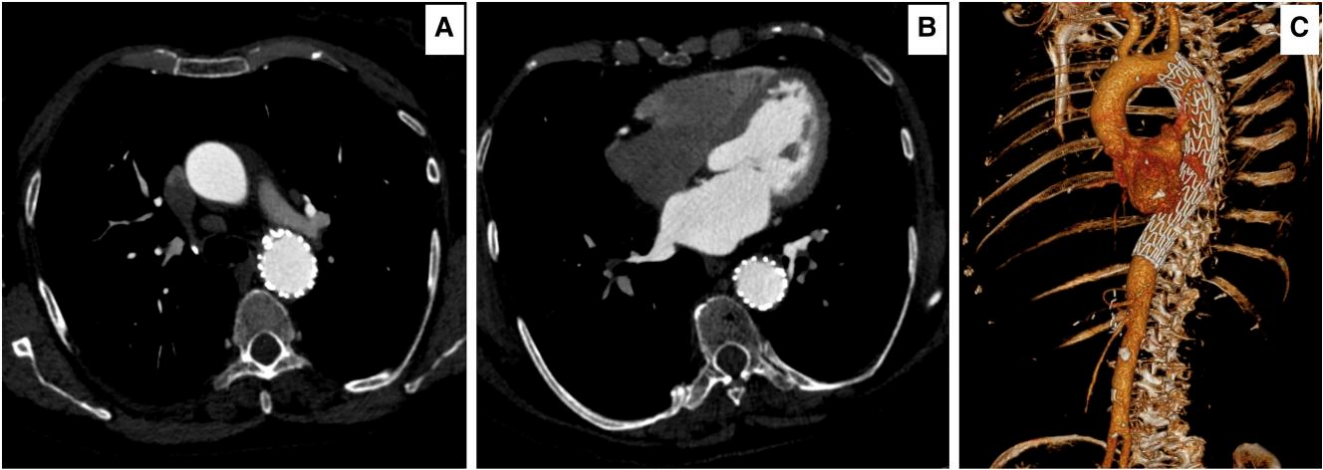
(*A*) A follow-up computed tomography angiography at 270 days post insult and thoracic endovascular aortic repair demonstrate total remodelling of the acute intra-mural haematoma with no evidence of endoleak and retrograde stent graft–induced new entry. (*B*) A follow-up computed tomography angiography at 270 days showing a completely ruptured acute intra-mural haematoma with a total resolution of the acute aortic catastrophe. (*C*) A three-dimensional reconstruction demonstrating patent celiac, both renal and the artery of Adamkiewicz (also arteria radicularis magna) at the level of the distal thoracic endovascular aortic repair, with no evidence of stent graft–induced new entry.
